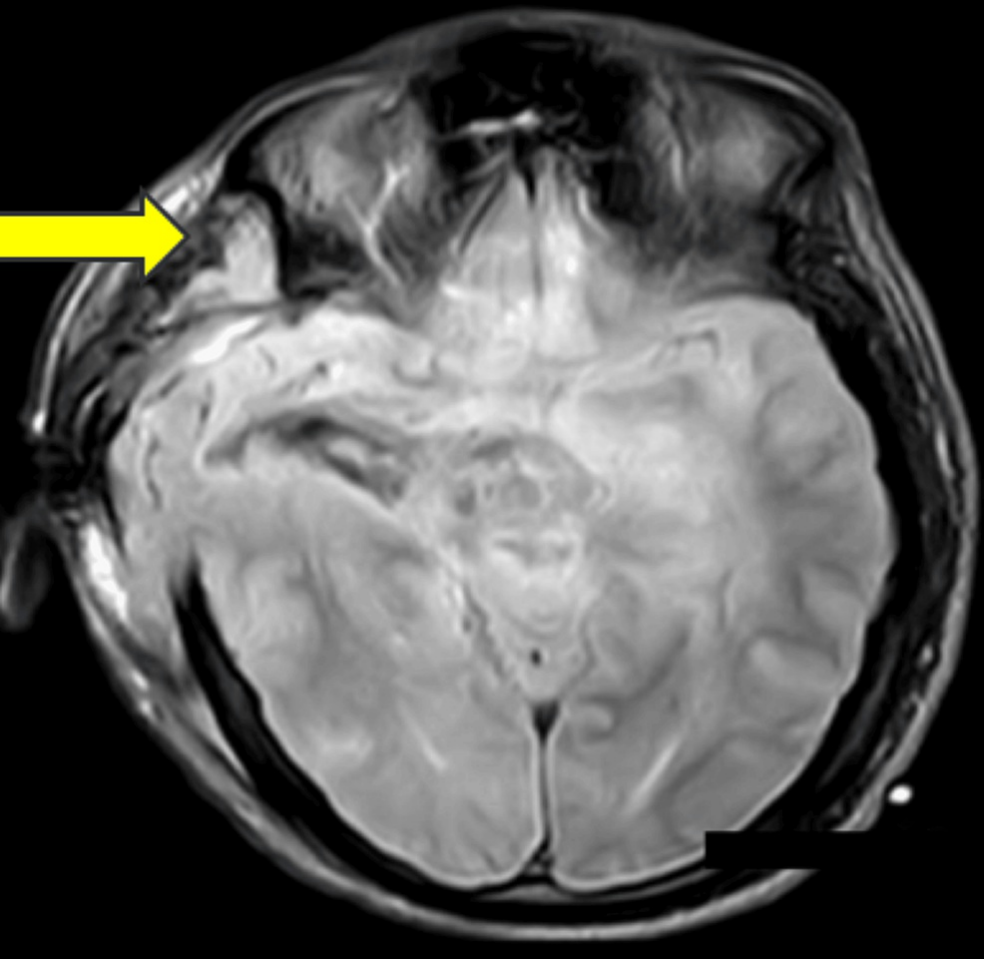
MRI of the brain revealed high-grade glioblastoma multiforme/astrocytoma indicated with a yellow arrow. MRI: magnetic resonance imaging
